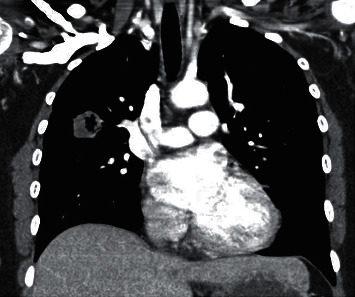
CT thorax showing the cavitary lesion in the right upper lobe of the lung.
